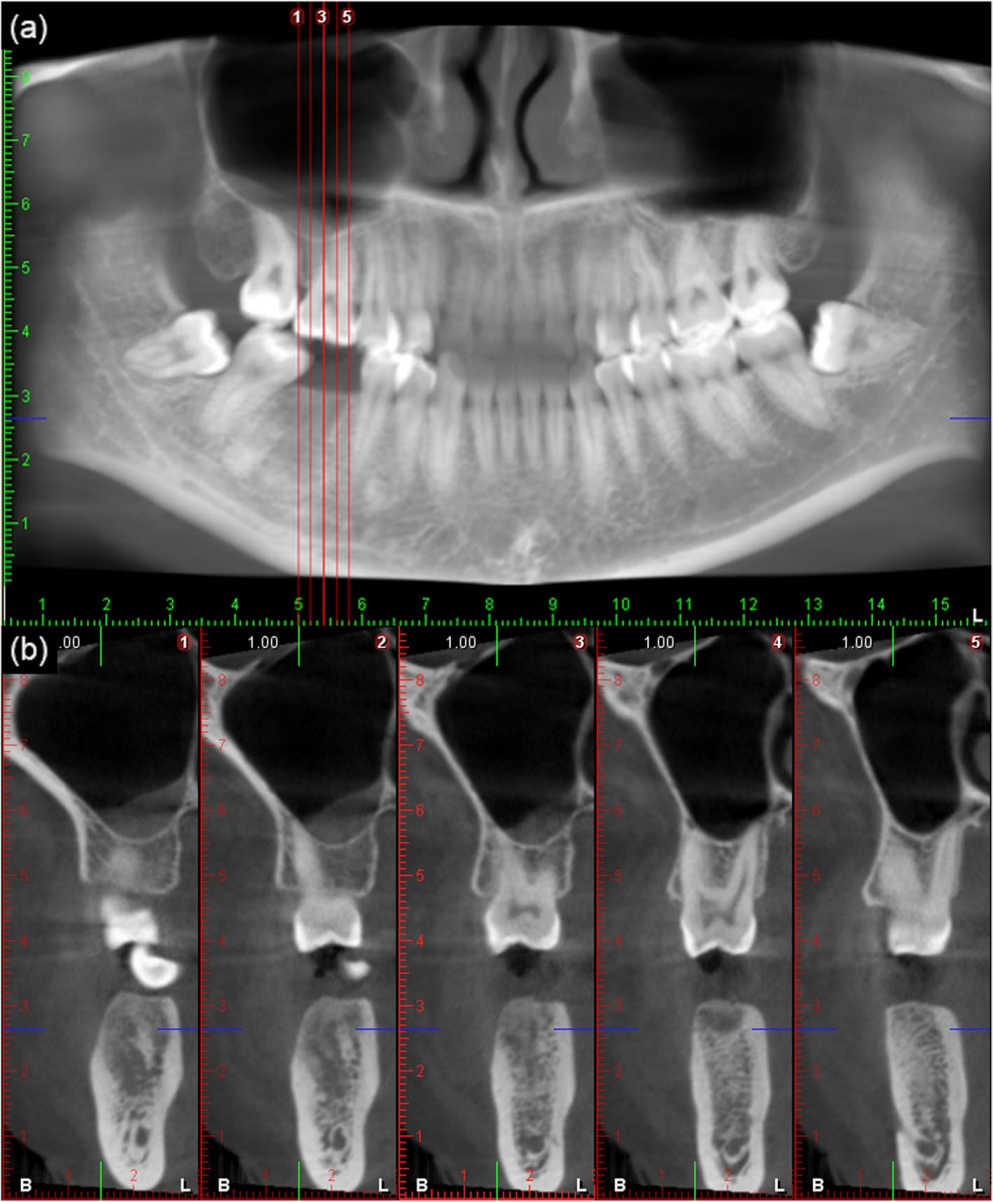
Presurgical CBCT of the patient. a Panoramic view; b Sagittal view of edentulous area
